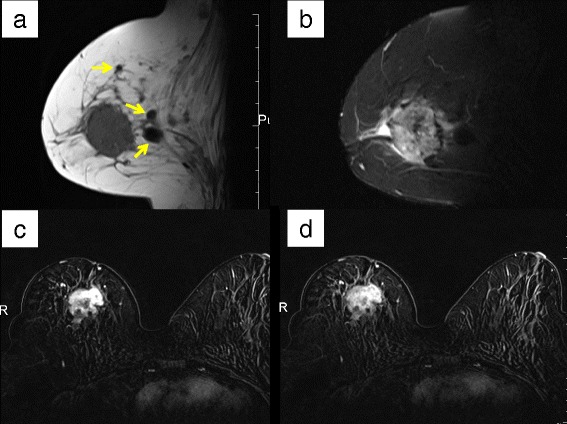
Breast magnetic resonance imaging (MRI). (a) T1-weighted MRI showed a low signal intensity (SI) mass (3.5 × 3.2 × 4.0 cm in size) that retracted the right nipple. Several oval-shaped low SI structures in adipose tissue and disruption of the pectoralis major muscle were also observed (arrows). (b) The mass showed intermingled high and low SI on T2-weighted images. (c, d) Heterogeneous early enhancement with central low intensity was noted on dynamic contrast-enhanced MRI (c: early phase, d: delay phase).
